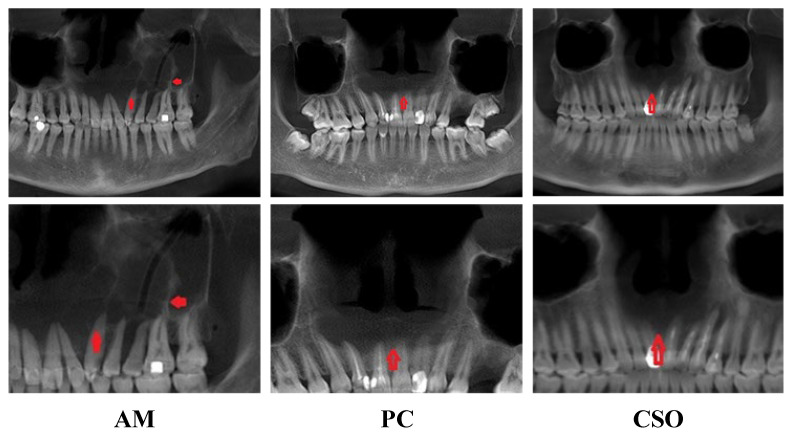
CBCT panoramic images of AM, PC, and CSO. The red arrows in the image indicate the lesion areas.
